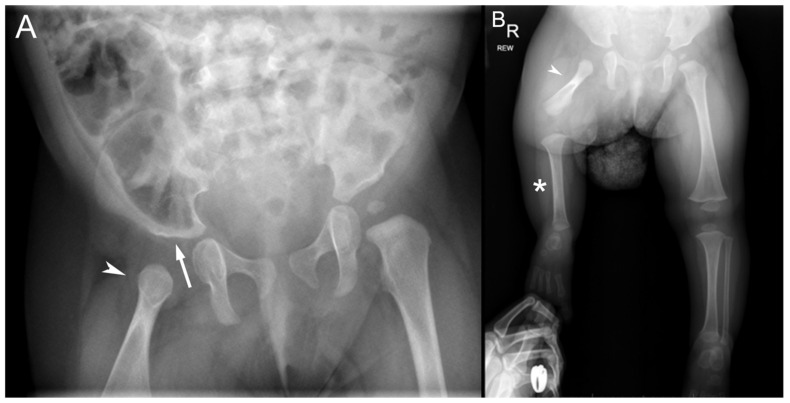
Radiographs at 8 months of age. (A) Pelvis radiograph shows a dysplastic right acetabulum (white arrow) and malformed proximal right femur (arrowhead). The proximal epiphysis, metaphysis, and lesser trochanter are unossified. (B) Bilateral lower extremity radiograph shows the hypoplastic right femur (arrowhead) consistent with PFFD, absent right fibula (*) consistent with fibular hemimelia. The contralateral left femur, tibial, and fibula are normal.
